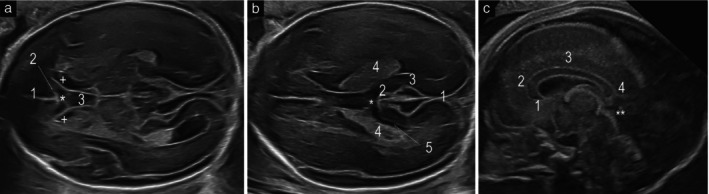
Transabdominal (a,b) and transvaginal (c) ultrasound images in a normal fetus at 22 weeks' gestation. (a) Image at the level of the anterior complex in the axial (transventricular) plane, showing interhemispheric fissure (1), callosal sulcus (2), cavum septum pellucidum (3), genu of the corpus callosum (
